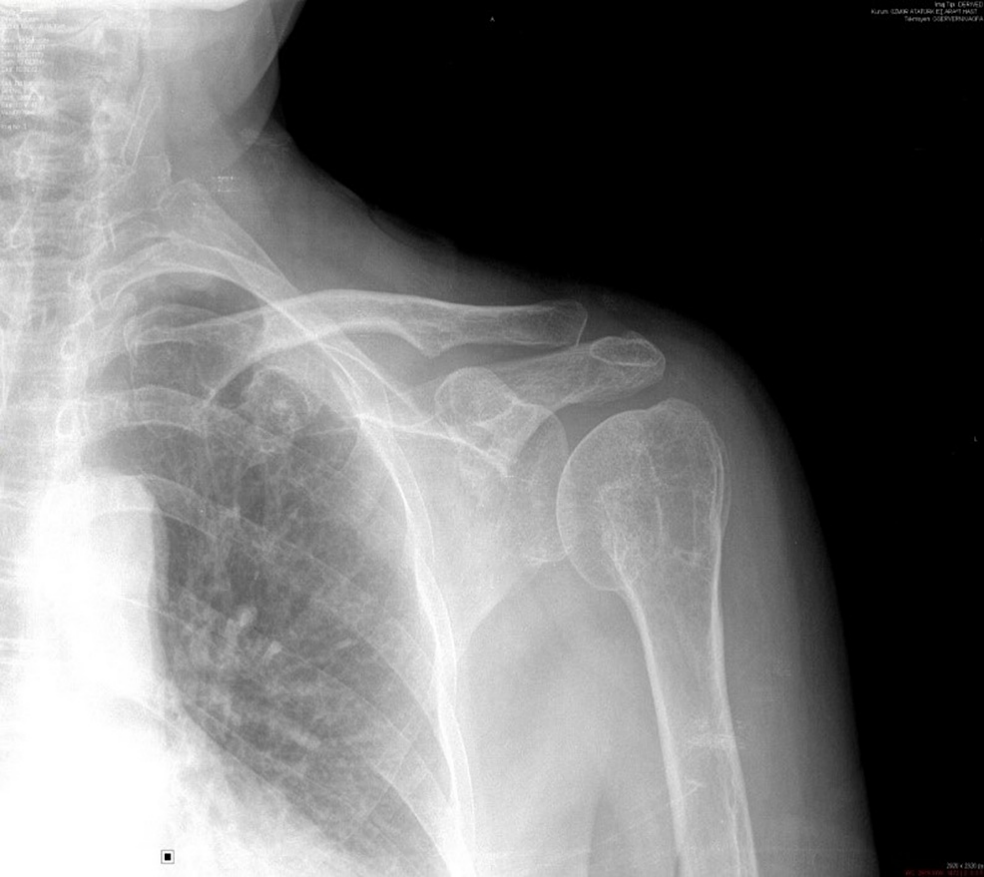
X-ray after conservative follow-up, full union achieved.
